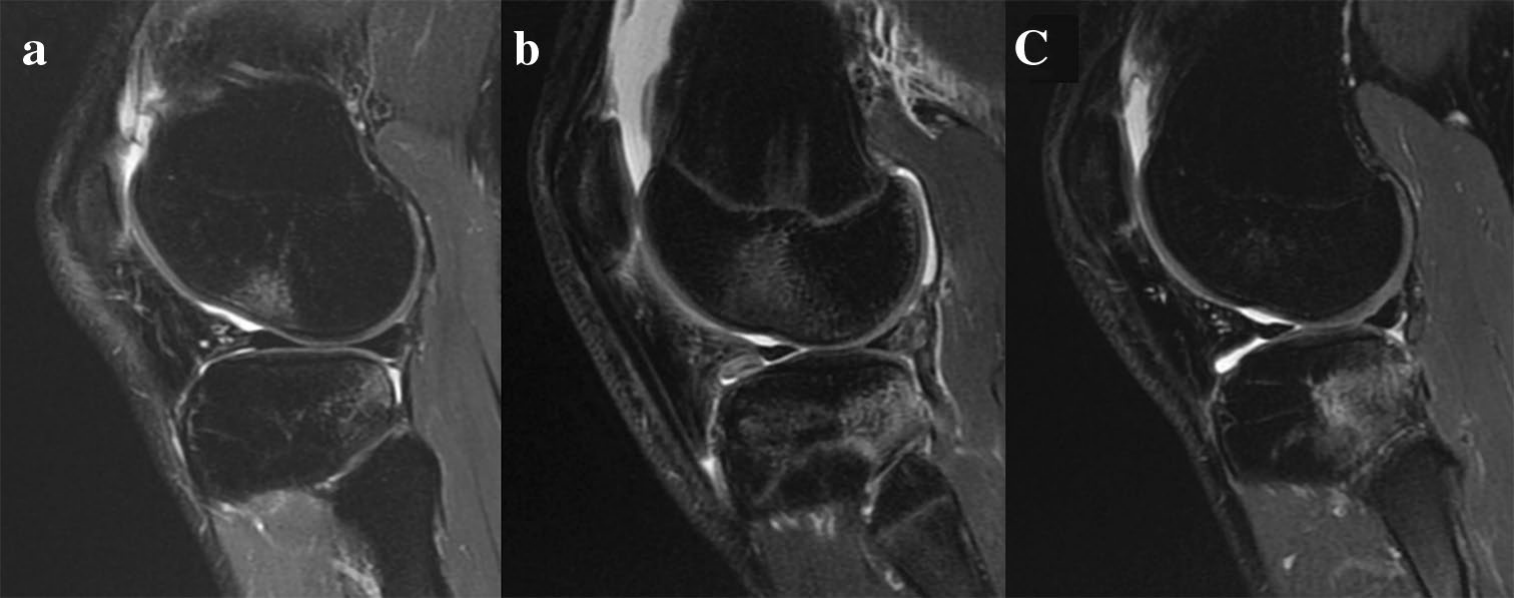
a–c MRI images of lateral BB from the case series*.* BB areas are predominantly located in posterior aspects of tibial plateau (inferiorly respect to articular cartilage and subchondral bone) and at the center of lateral femoral condyle
